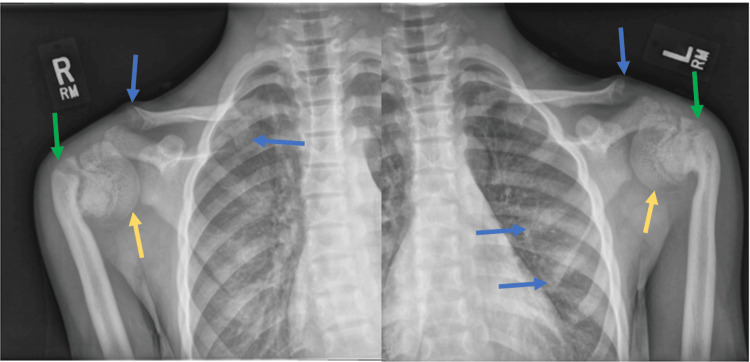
Plain films of bilateral shoulders Plain films of bilateral shoulders in pseudohypoparathyroidism demonstrating subchondral resorption of the ends of the clavicles and ribs (blue arrows), band lucencies of proximal humeral metaphyses with secondary vara deformity (green arrows), and inferior subluxation of the humeral heads (yellow arrows).
